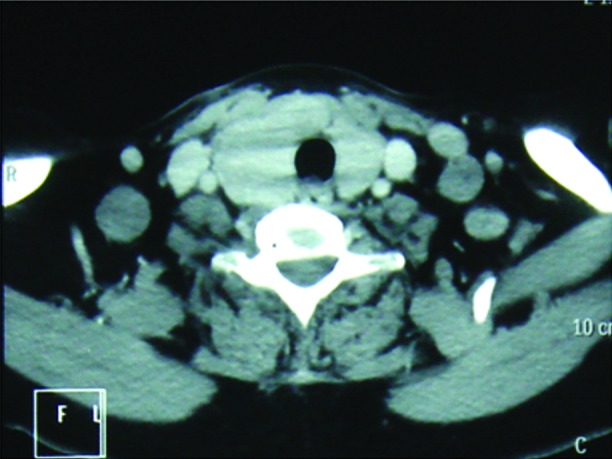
Computed tomography (CT) scan of the neck showing bilateral supraclavicular lymphadenopathy.
